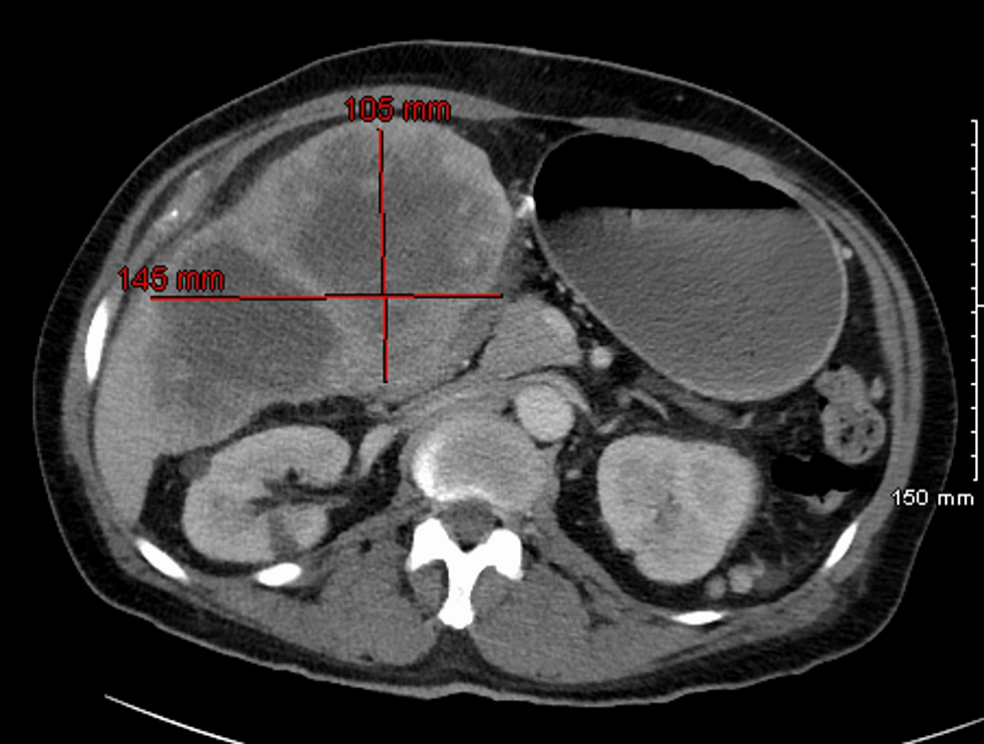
CT Abdomen (axial view) showing a large heterogeneous mass which appears continuous with the gallbladder and liver. The mass likely extends from the gallbladder fundus into the liver parenchyma. The mass is peripherally enhancing with central hypoenhancement having multiple patchy areas of enhancement. The mass measures 105 x 145 x 154 millimeters in AP, transverse, and craniocaudal dimensions. CT: computed tomography.
